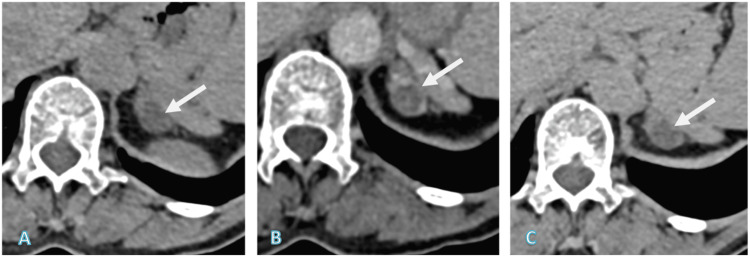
Left adrenal adenoma: adrenal protocol axial CECT image shows a small, well-defined left adrenal lesion (white arrows) with low unenhanced attenuation (A), showing moderate enhancement (B) and rapid contrast washout (C), consistent with lipid-rich adenoma. CECT = contrast-enhanced CT
